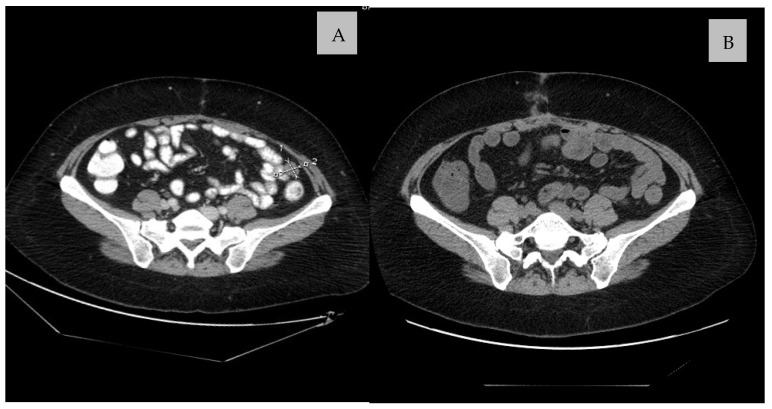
CT scan before (A) and after (B) resection of residual metastatic lesion in the abdominal cavity in young women with GIST with *KIT* exon 9 deletion mutation arising from the small intestine, after treatment with TKIs.
